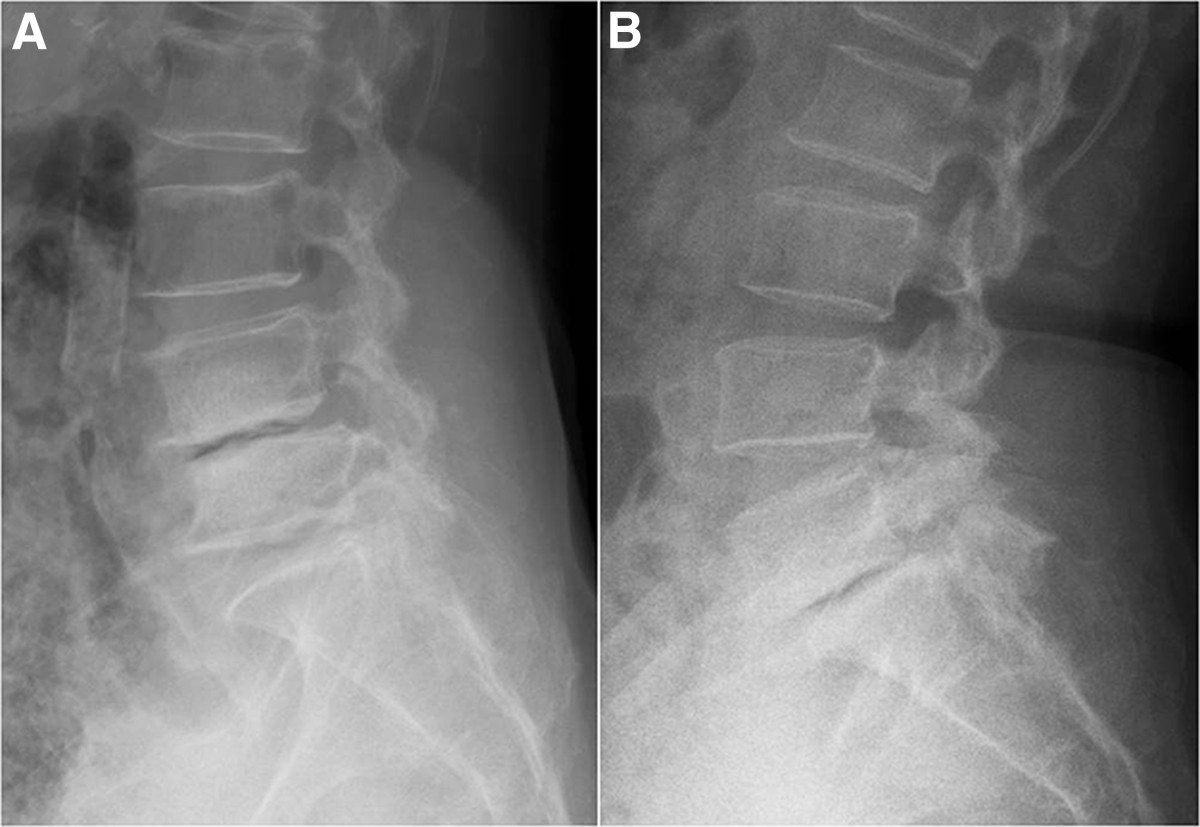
Radiography of degenerative and spondylolytic spondylolisthesis.
